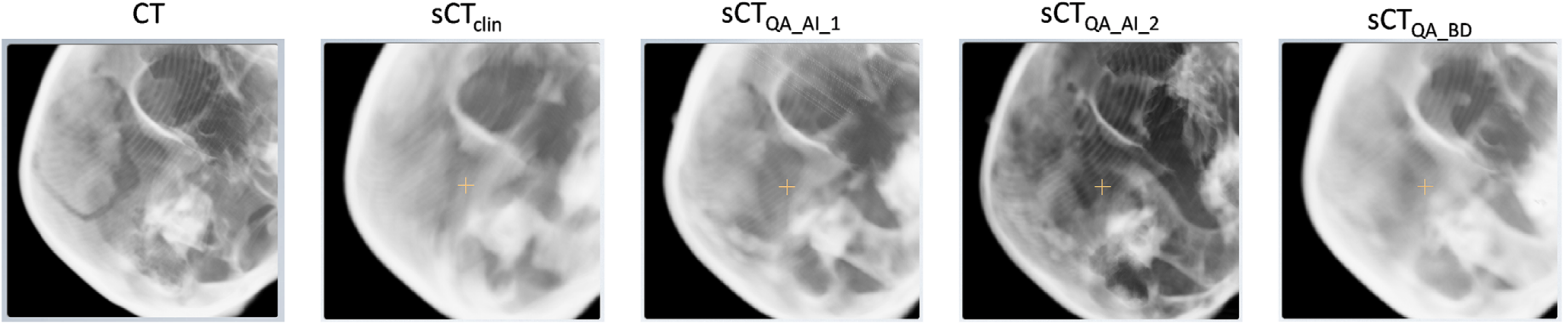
Example of DRR and sDRR. sDRR, synthetic digitally reconstructed radiographs.
